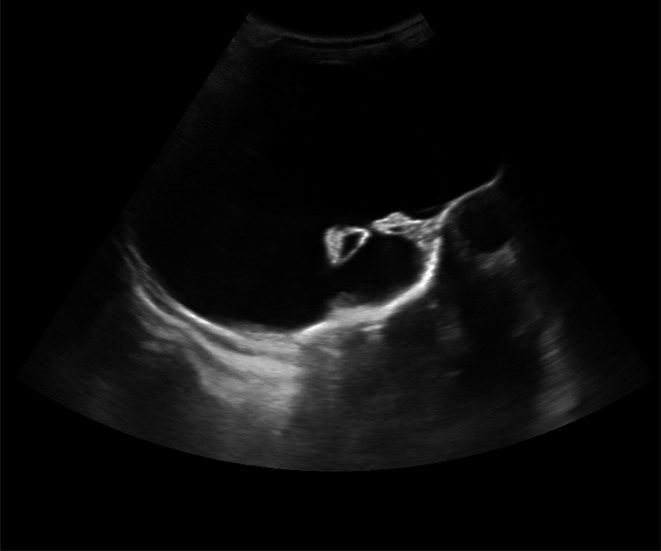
Cranial ultrasound sagittal view with non‐visualization of bilateral cerebral hemisphere which is replaced by fluid.
